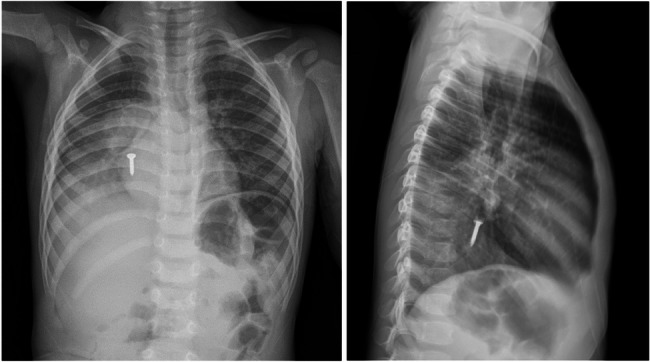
Chest X-ray (upright and left lateral) showing distal foreign body obstruction of a tiny nail in a 2-year-old boy
